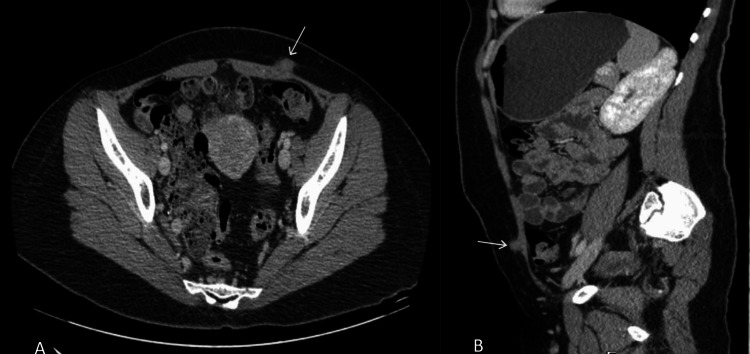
Contrast-enhanced CT of the abdomen showing a soft tissue mass in the left rectus abdominis muscle in axial (A) and sagittal (B) sections.
